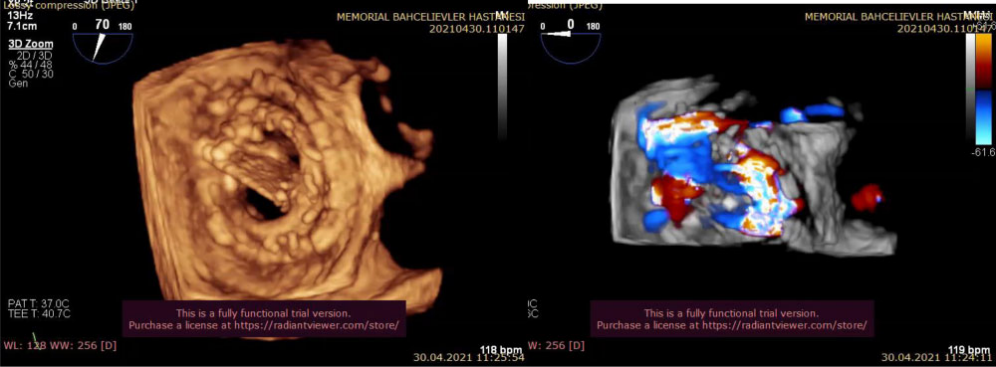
The dehiscence of the prosthetic valve and the paravalvular regurgitation with colour Doppler.
